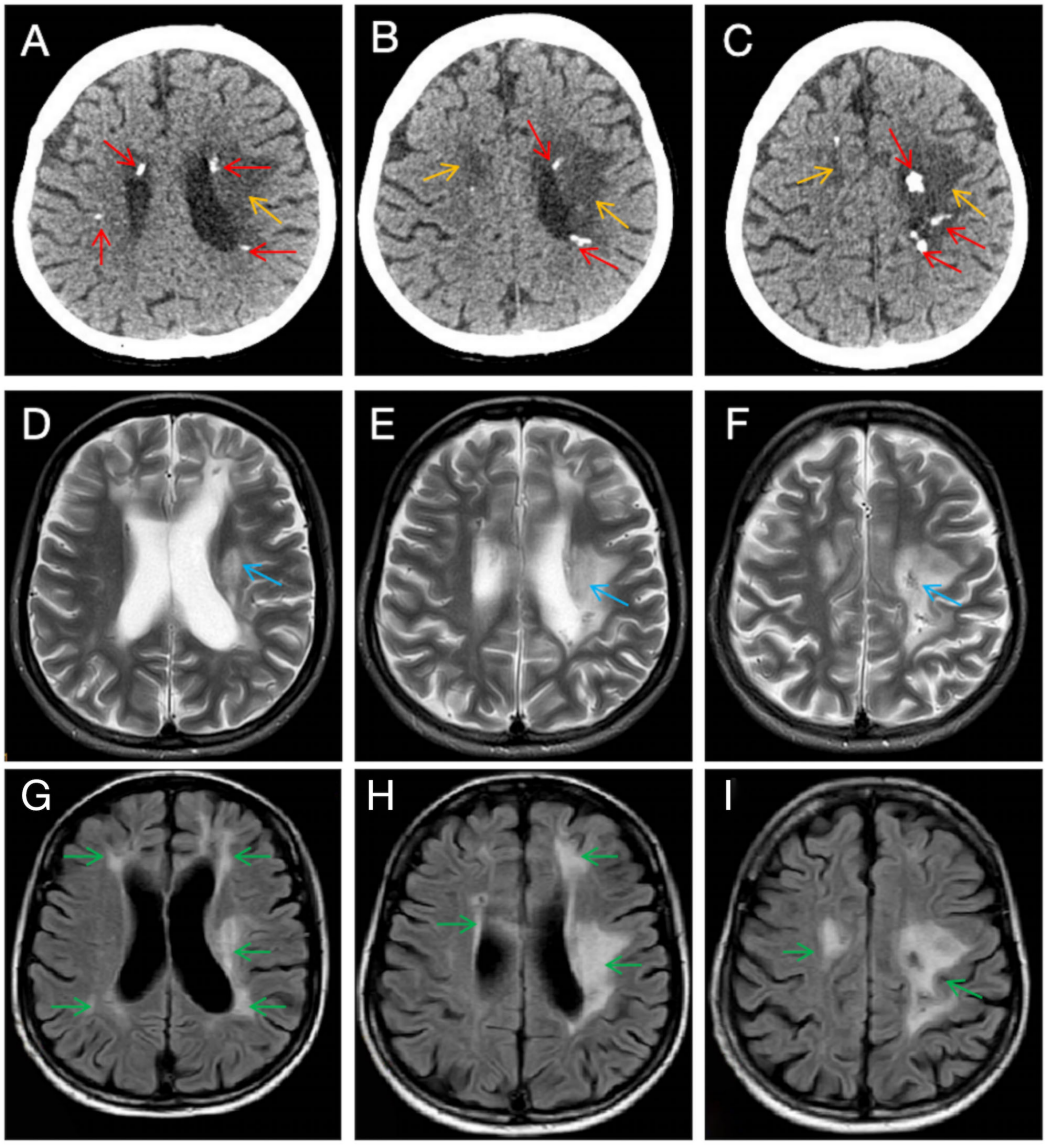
Neuroimaging features of the patient. (A–C) Non-contrast head CT scan reveals extensive hypodense lesions in the periventricular regions [orange arrows, (A, B)] and centrum semiovale [orange arrow, (C)], accompanied by multiple calcifications [red arrows, (A–C)]. (D–F) T2-weighted MRI shows non-specific T2 hyperintense white matter lesions (blue arrows) and cerebral atrophy. (G–I) FLAIR MRI demonstrates extensive, asymmetrical, confluent lesions involving the periventricular regions, frontal, and parietal lobes (green arrows).
